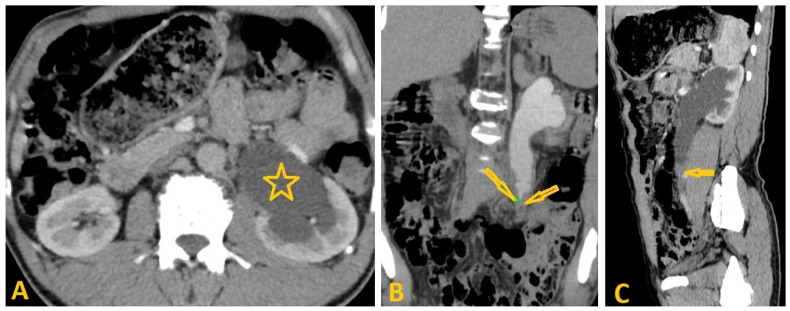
Selected non-contrast computed tomography axial images of a 45-year-old male patient presenting with left abdominal pain show left kidney delayed excretion of contrast for 30 min with moderate left hydroureteronephrosis (star on the axial image (A)) due to circumferential ureteral wall thickening at the middle part (arrows on coronal reconstruction image (B)), causing severe ureteric liminal narrowing measuring 6 cm in length (arrow on sagittal reconstruction (C)). In addition, there is 9 × 4 mm oval-shaped left para-aortic enlarged lymph node. This case was diagnosed as left ureteric transitional cell carcinoma in a proven case of chronic Schistosomiasis.
